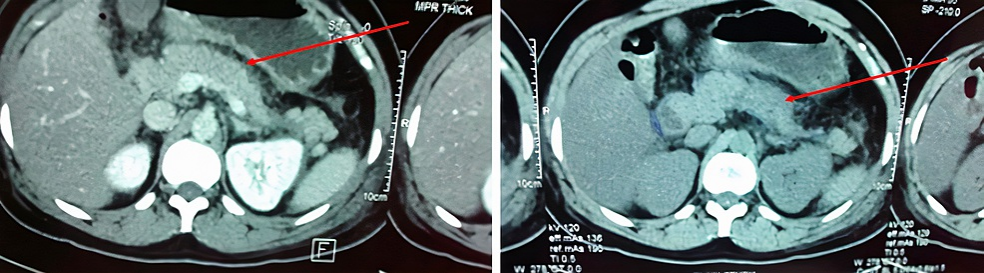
Contrast-enhanced CT (CECT) abdomen of patients 2A and 2B: CECT abdomen showing bulky pancreas with peripancreatic inflammatory stranding and fluid collection suggestive of acute pancreatitis
